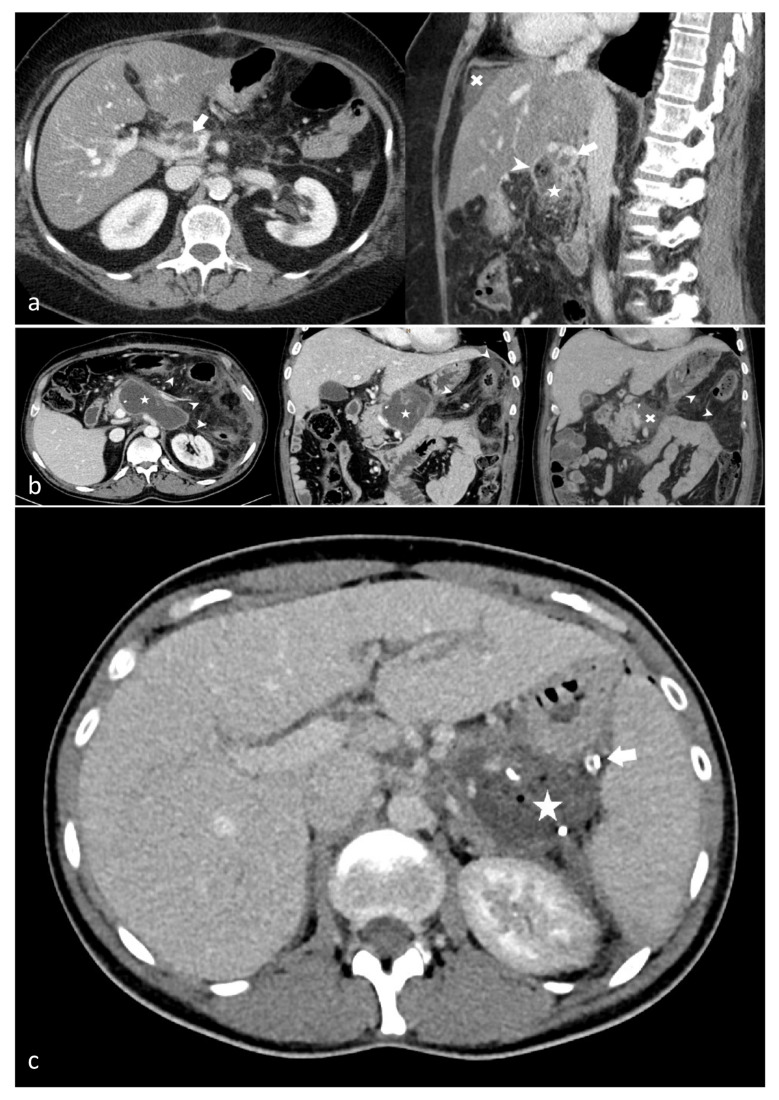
(a–c). Exemplary findings in PPAP scans: (a) Arrow: Portal vein thrombosis in the CT scan on 10th POD; Arrowhead: Drainage; Star: Remnant pancreas; Cross: Perihepatic ascites; (b) Star: Pseudocyst in the CT scan several weeks post-operation; Arrowheads: Mesenteric fat stranding; Cross: Peripancreatic fluid collection in the first postoperative CT scan; (c) Star: Air-containing peripancreatic collection in the first postoperative CT scan; Arrow: Drainage.
